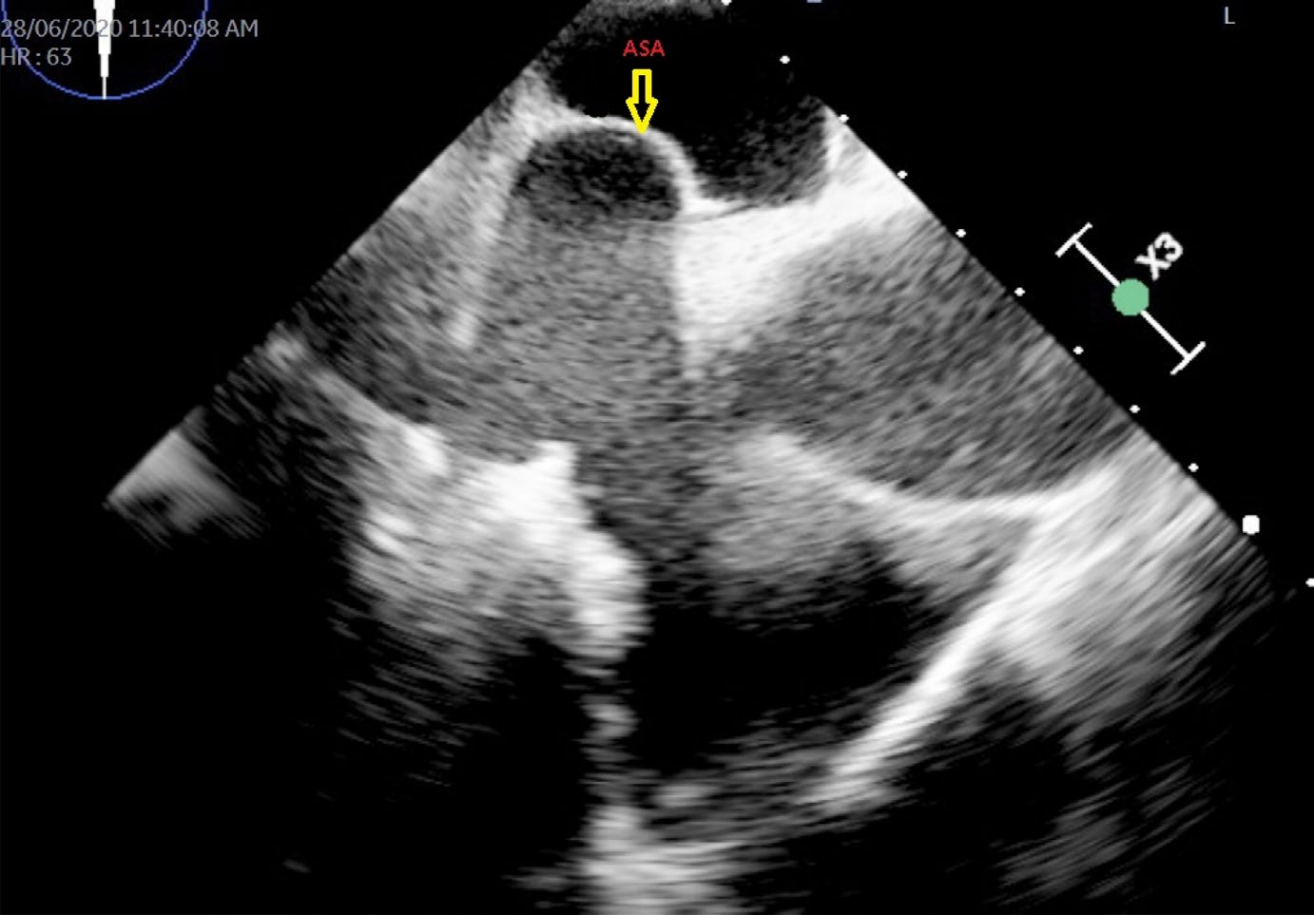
Transesophageal echocardiogram and yellow arrow showing atrial septal aneurysm (ASA)
